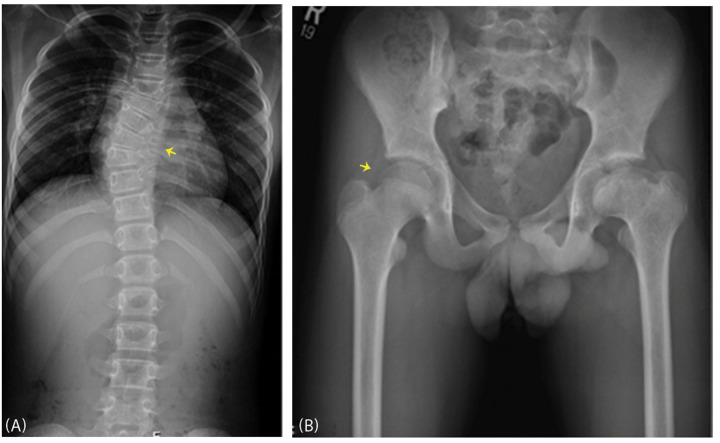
Radiographic skeletal findings in Patient 3 with KBG syndrome. (A) Anteroposterior thoracolumbar spine x-ray demonstrating right thoracic scoliosis with a suspected butterfly vertebra in the mid-thoracic region (yellow arrow). (B) Pelvic x-ray showing bilateral femoral head widening, more pronounced on the right side, with associated areas of sclerosis (yellow arrow).
